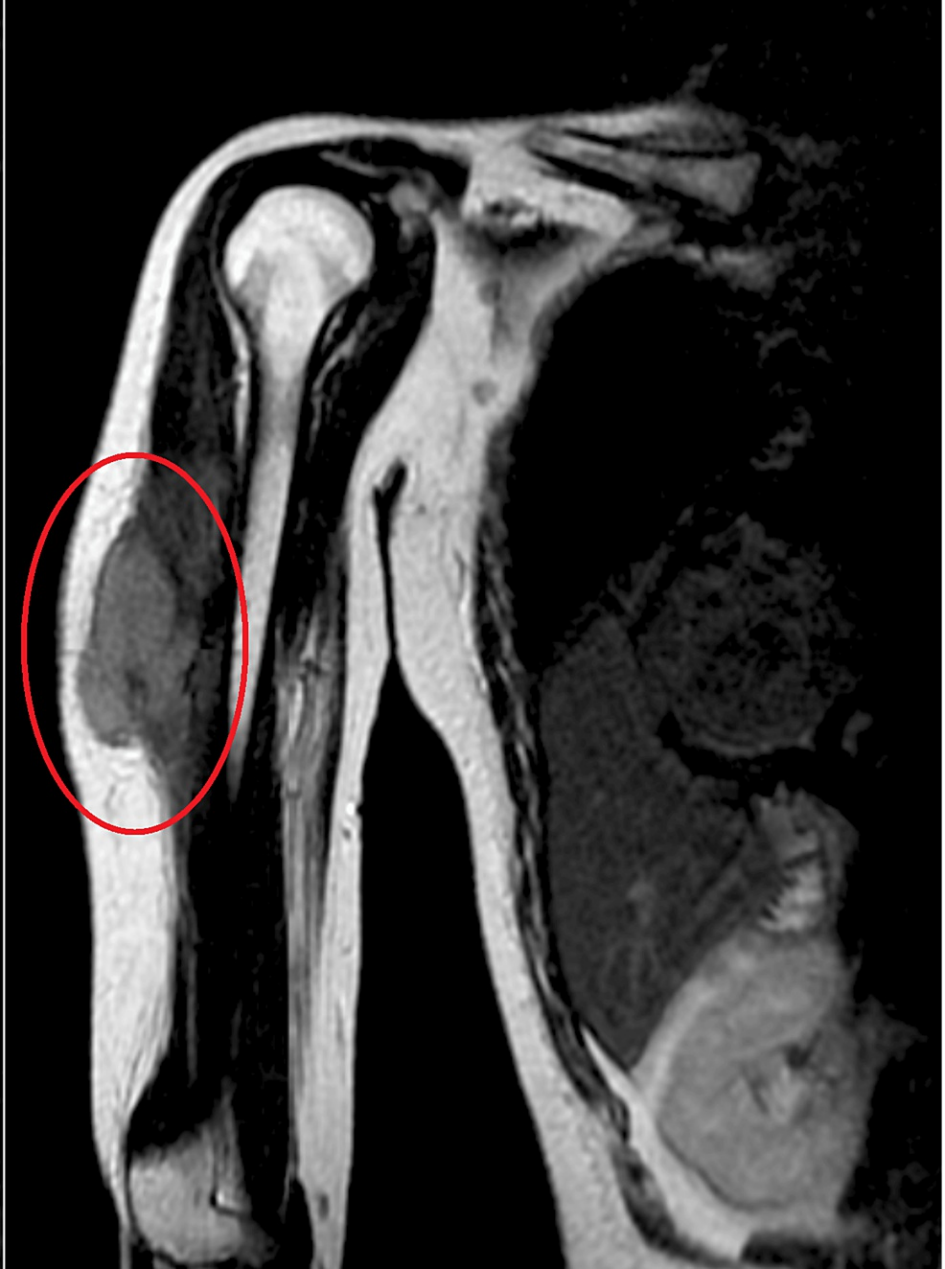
MRI of the right arm The red circle region shows a well-defined mass lesion involving the distal deltoid muscle and the adjoining lateral head of the triceps muscles.
